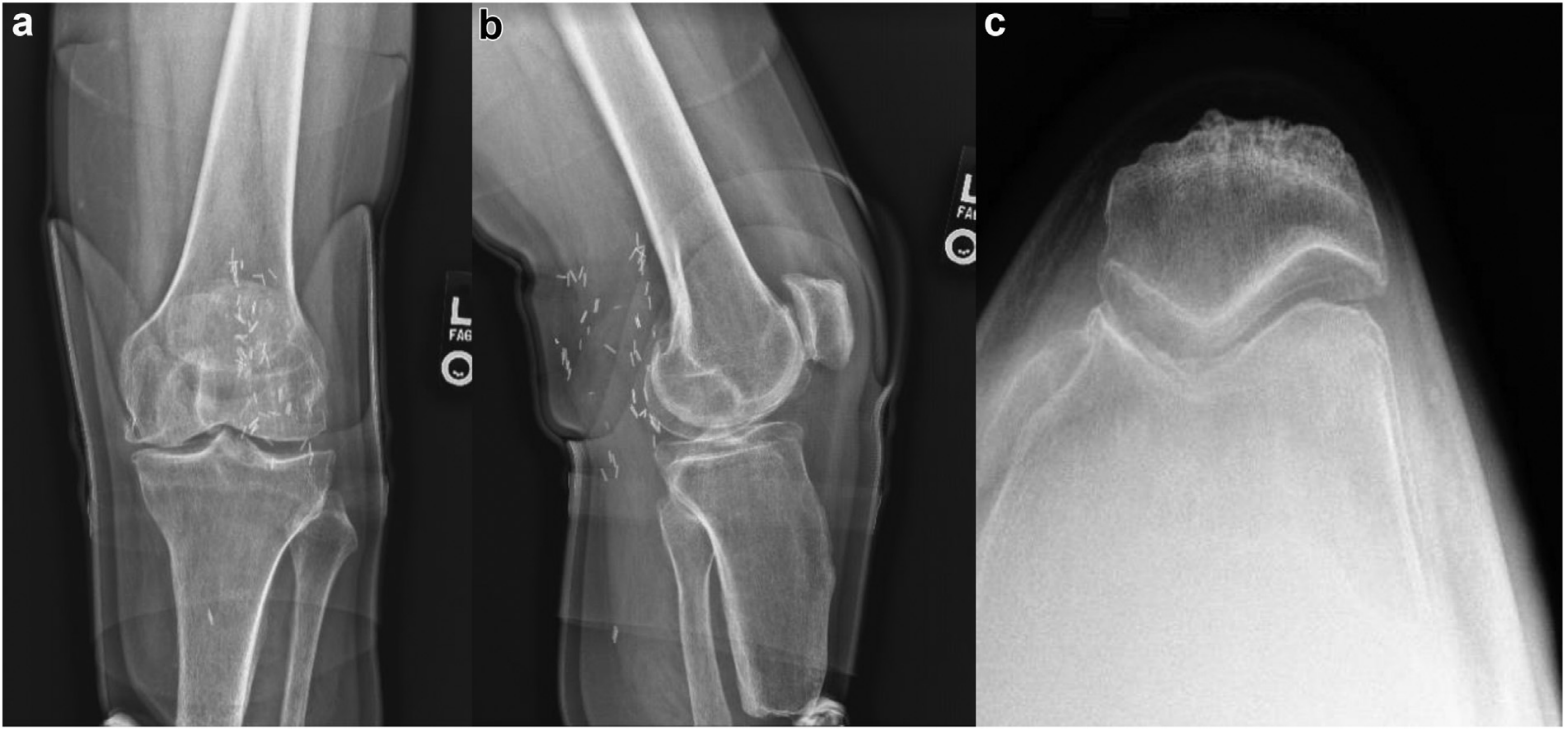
Preoperative radiographic imaging of the right knee: (a) anteroposterior, (b) lateral, and (c) sunrise view.
